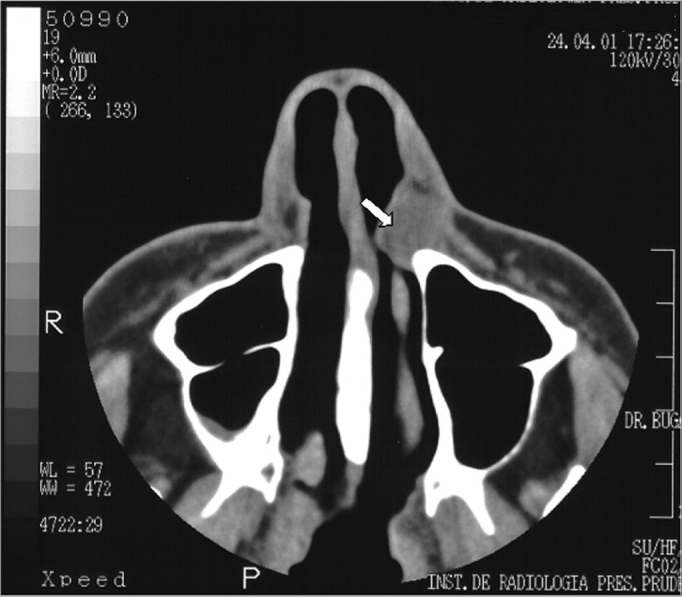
CT image (axial section) showing a heterogeneous mass suggesting soft tissue in the left nasal fossa (arrow).
